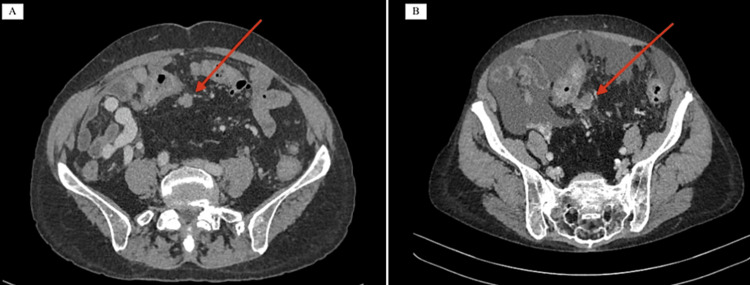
CT imaging from 2016 (A) demonstrating an unreported non enhancing mass in the sigmoid mesentery, CT imaging from 2021 (B) demonstrating progression of the mass with calcifications, and ascites CT: Computed Tomography
